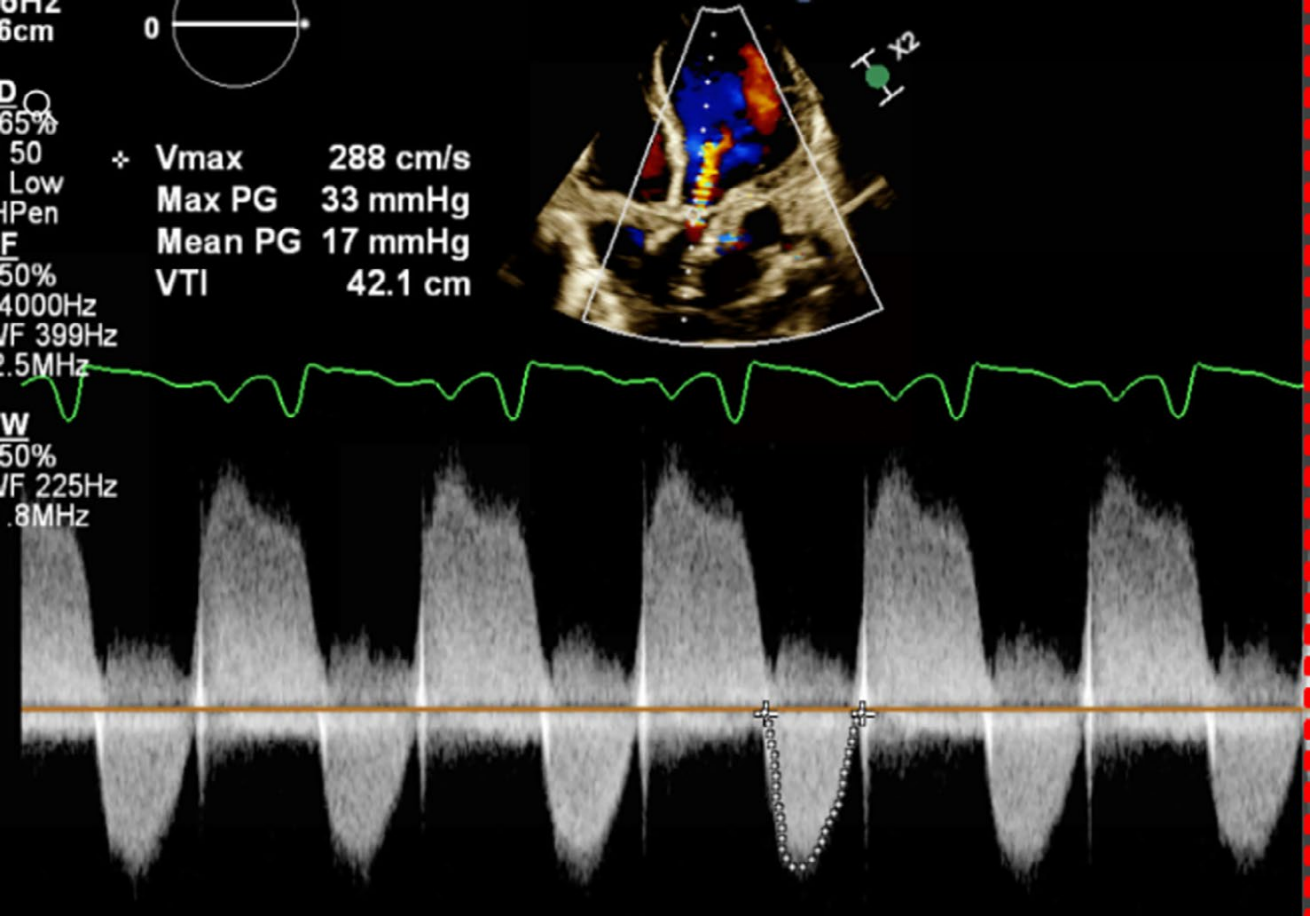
CW Doppler showing severe AS in the 4‐chamber view.
